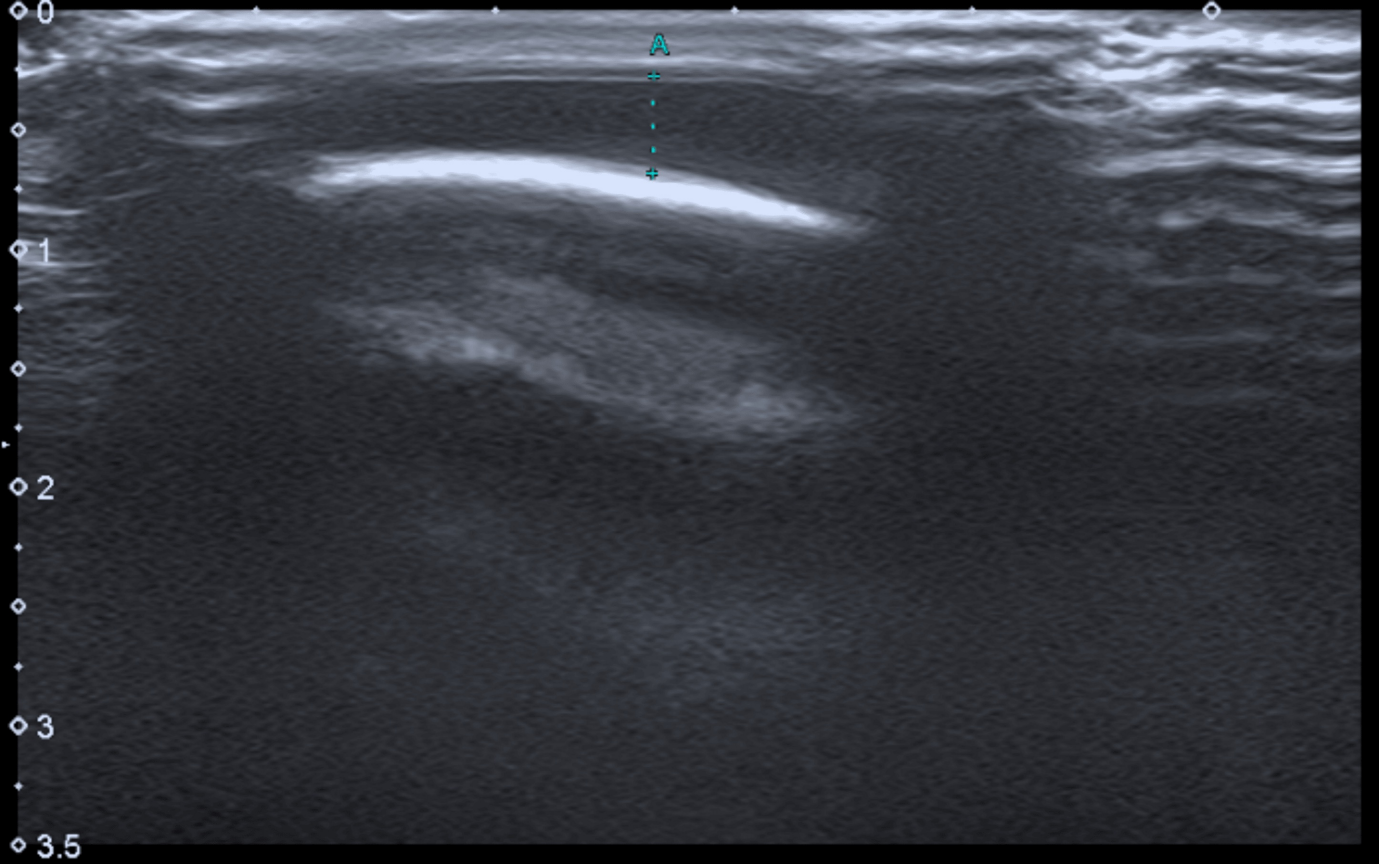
Cranial ultrasound - a small anechoic collection is identified (A), with simple ultrasound characteristics, not organized, in a subaponeurotic location.
